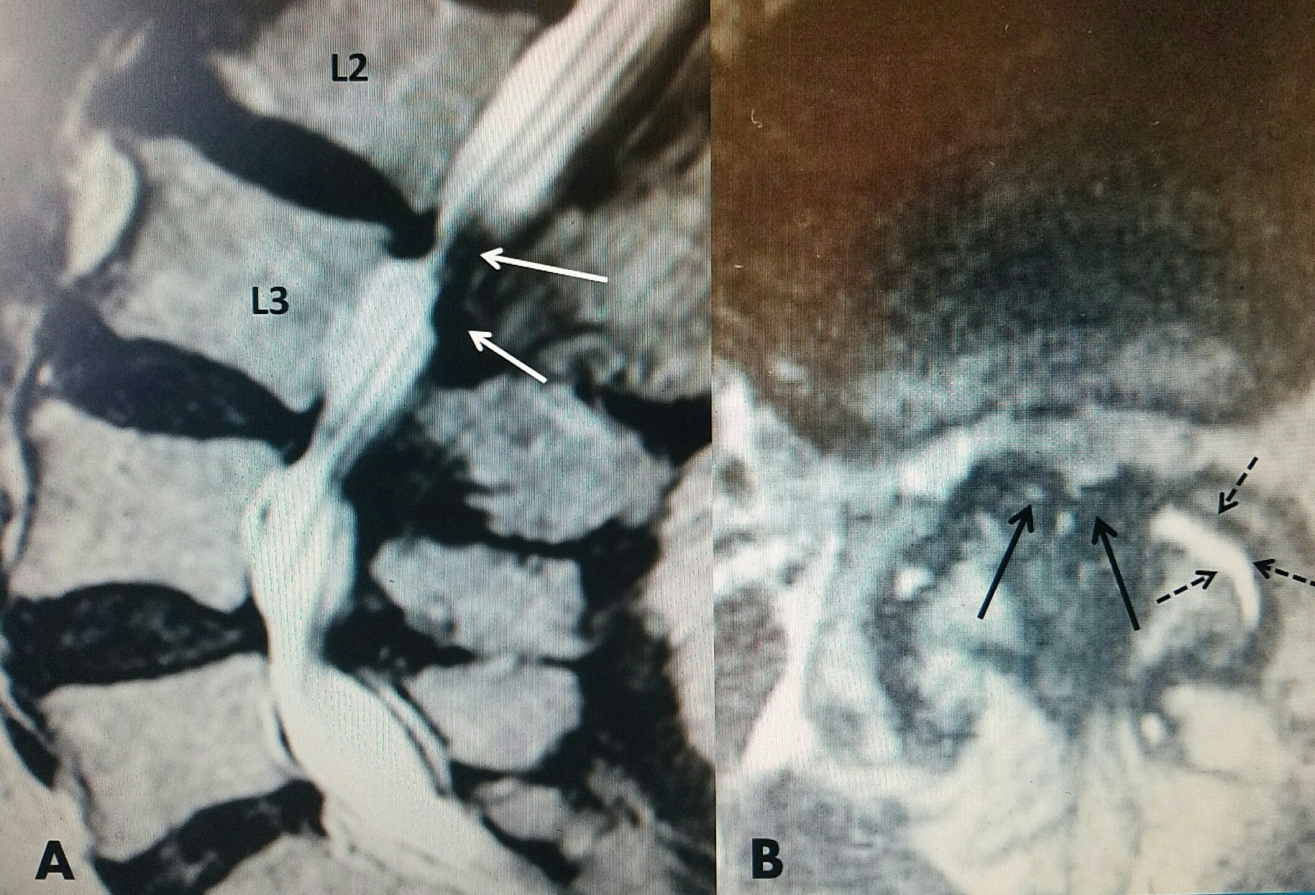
MRI showing multilevel stenosis with L2-3 ligamentous stenosis A: T2 sagittal MRI shows severe posterior L 2-3 ligamentous compression (white solid arrows) with a ventral L 2-3 disc. There is associated lesser degree of stenosis at L 3-4 and L 4-5. B: T2 axial MRI shows marked ligamentum flavum hypertrophy (black solid arrows) and marked fluid in the left facet joint (dashed arrows). MRI: Magnetic resonance imaging.
